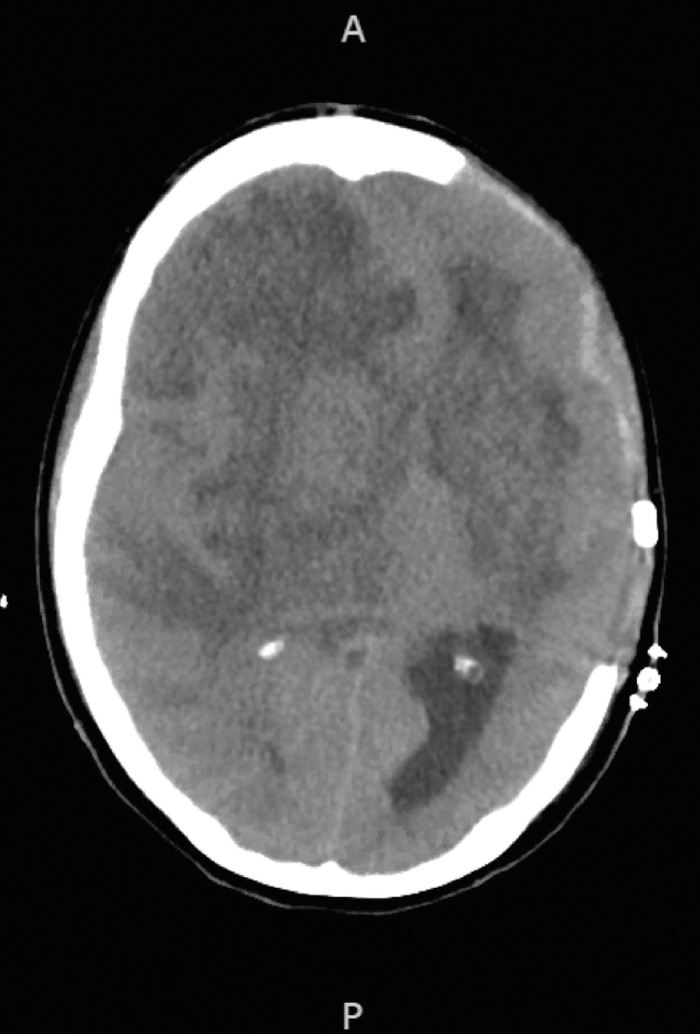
Computed tomography head after left decompressive hemicraniectomy.
